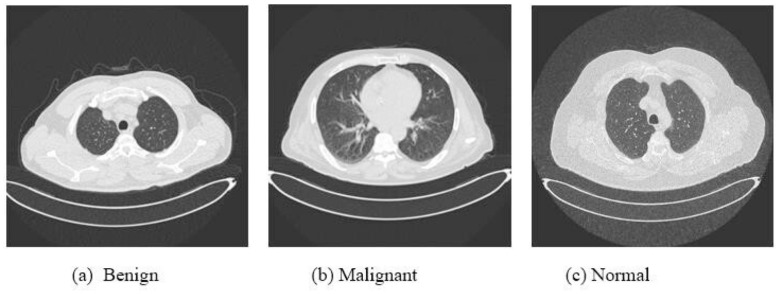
Representative CT scan samples from the three-class lung cancer dataset used in this study. (a) Benign: early-stage tumor presenting as a small, well-circumscribed nodule without evidence of malignant invasion. (b) Malignant: advanced cancerous tumor with irregular margins, potentially indicating spread beyond the primary site. (c) Normal: healthy lung parenchyma without visible nodules or abnormalities. All images are 2D axial CT slices processed at 512 × 512 resolution.
